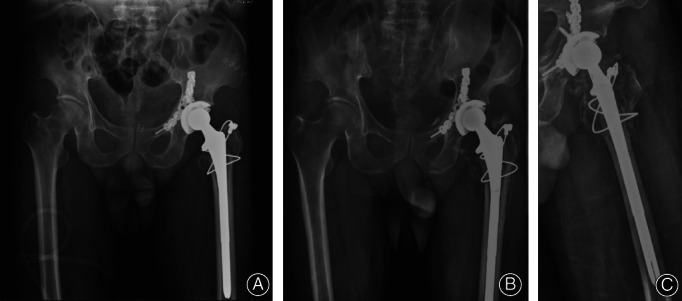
(A) Immediate radiograph after total hip replacement (THR). (B–C) Anteroposterior and lateral radiographs at the 2‐year follow‐up.
